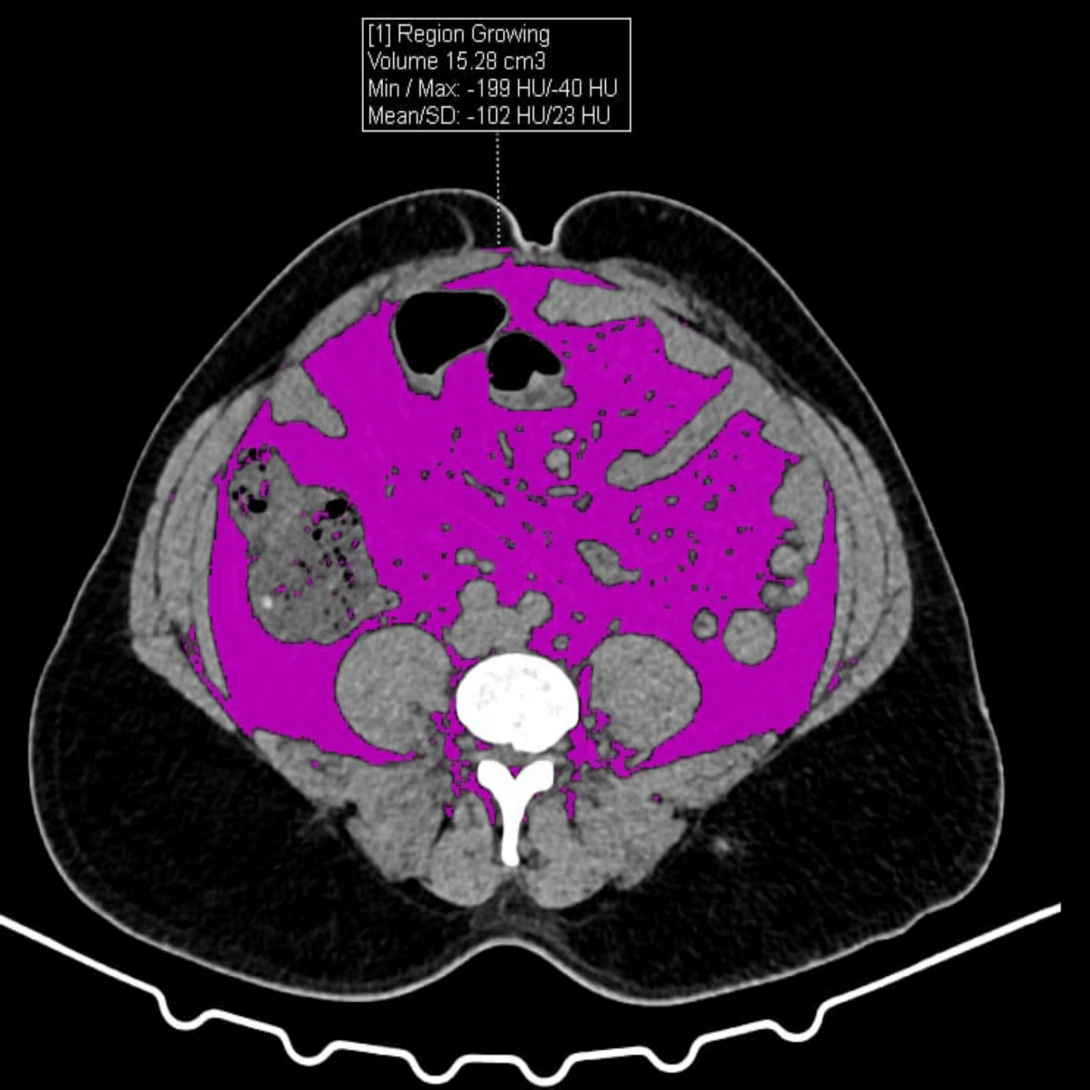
VAT calculations by CT within the ROI (region of interest) VAT: Visceral Adipose tissue
